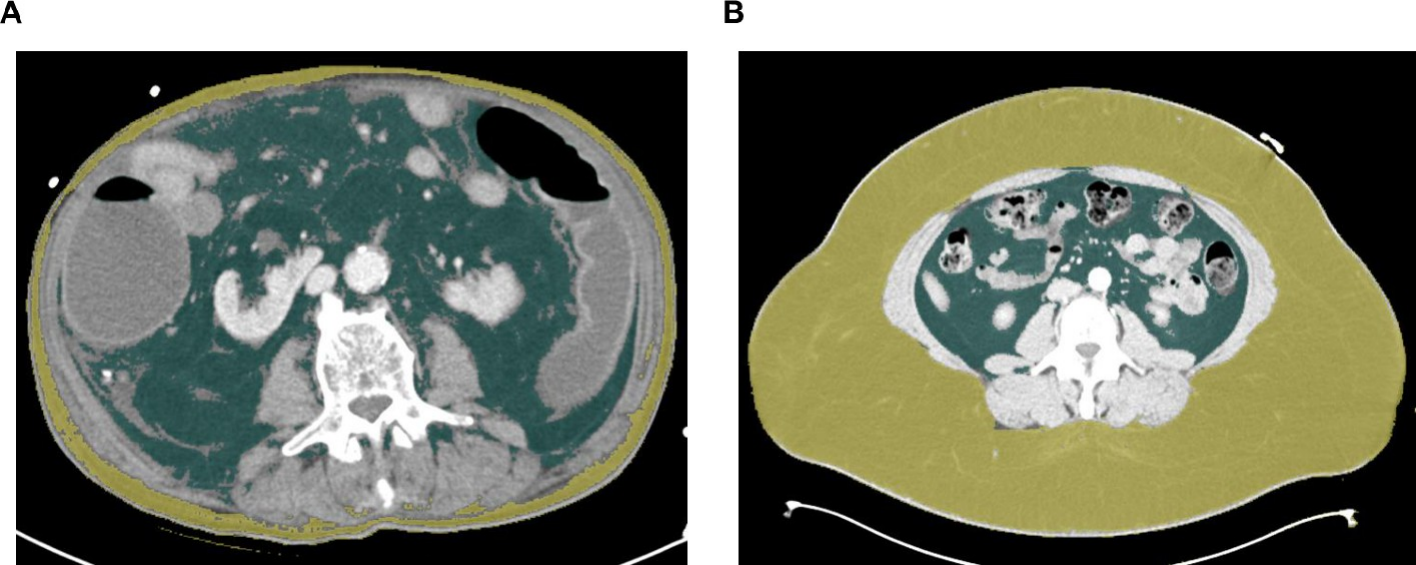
Assessment of visceral and subcutaneous adipose tissue distribution. The visceral and subcutaneous adipose tissue areas were segmented at the center plane of the 3^rd^ lumbar vertebra on axial CT-scans using a semi-automatically segmentation tool (3D slicer) [13]. (A) An exemplary scan of a patient with excessive visceral (green area) and low subcutaneous (yellow area) adipose tissue is depicted. (B) An exemplary scan of a patient with low visceral (green area) and excessive subcutaneous (yellow area) adipose tissue is depicted.
